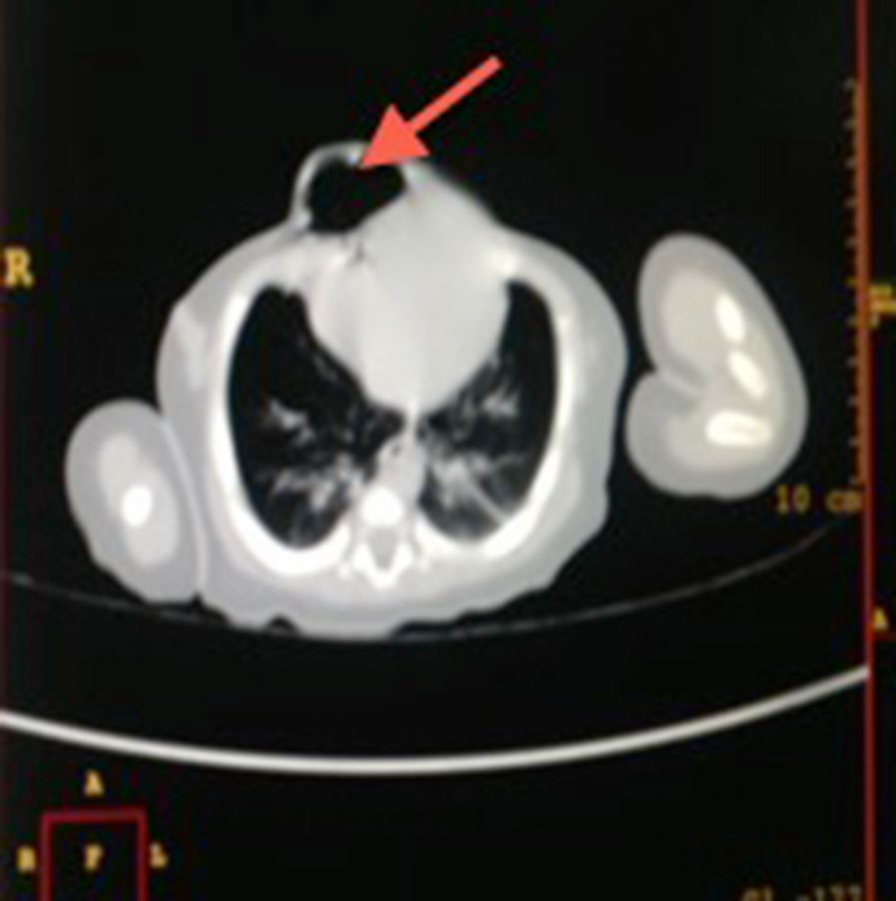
Axial CT scan of the patient showing the heart bulging though the anterior abdominal wall defect proximal to air filled bowel loops.
